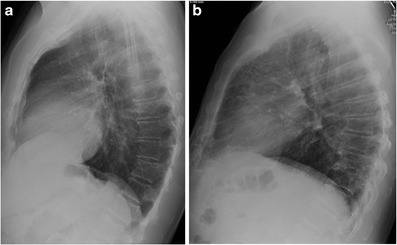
63-year-old asymptomatic man with a normal radiograph (a) showing fine linear opacities in the retrosternal clear space that taper before reaching the sternum. In contrast, coarse reticular opacities form a mesh and extend to the sternum (b) in a 67-year-old man with an unclassified pulmonary fibrosis (CT not shown)
